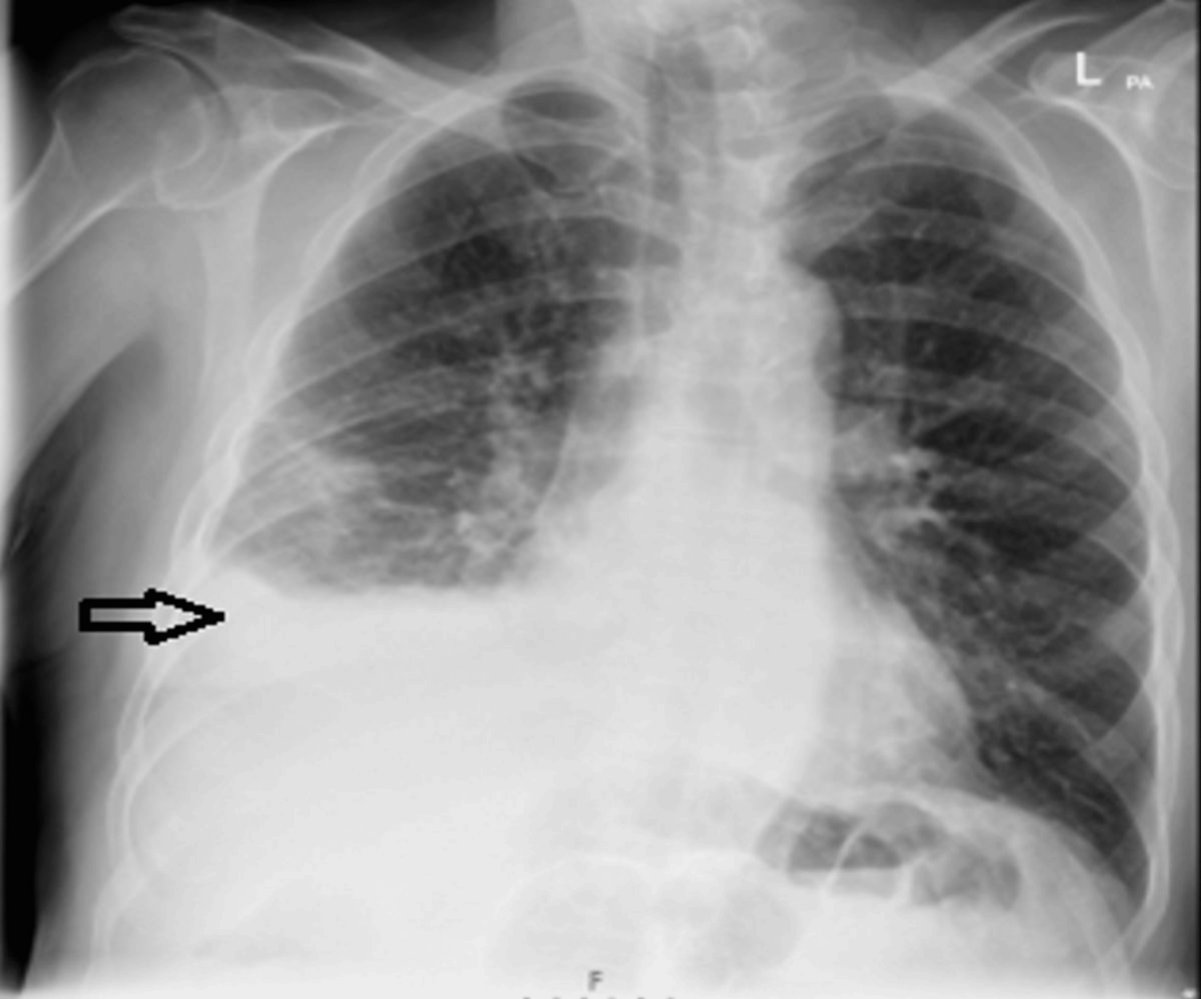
The chest X-ray showed increased consolidations in the right lower and middle lung zones, as well as increased right-sided pleural effusion.
